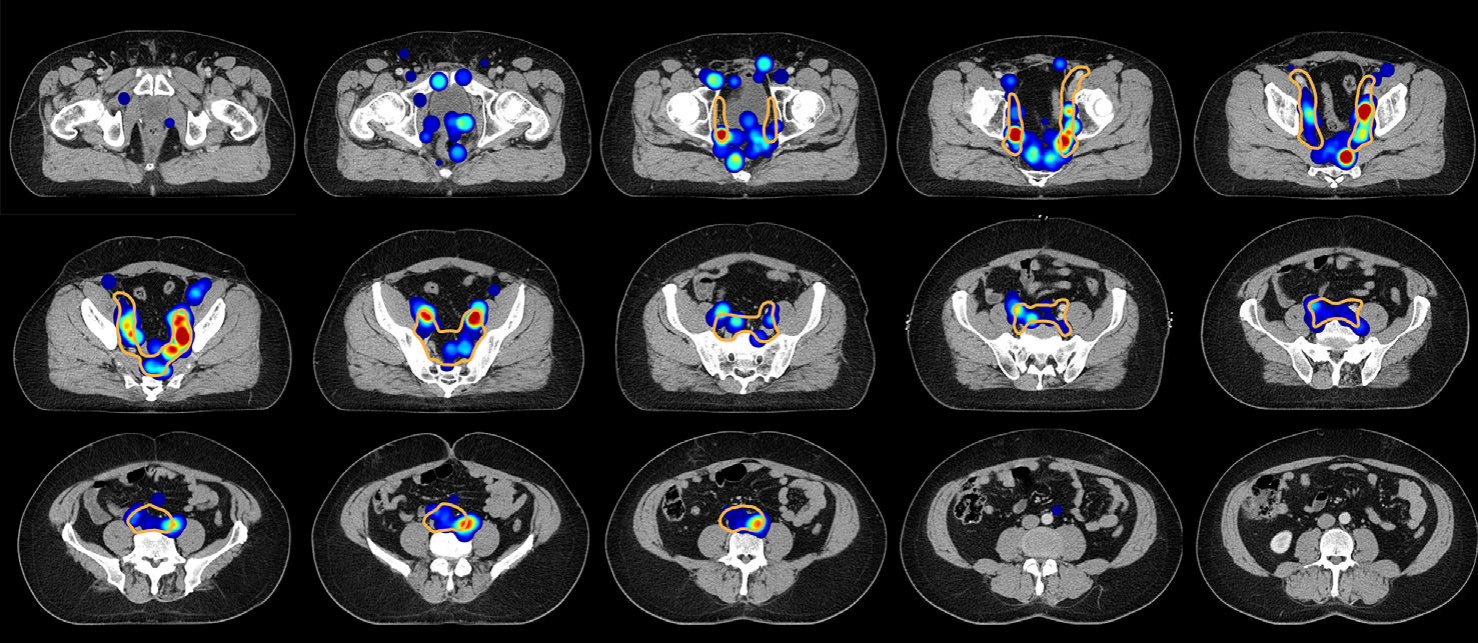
Axial slices of the created CT atlas visualize the estimated three-dimensional probability density function of pelvic nodal metastases for the whole cohort in a common reference CT. Weighting was applied so that each patient case contributed equally to the estimate irrespective of the amount of positive nodes. Slice spacing is 15 mm. Solid orange contour: Clinical target volume defined according to the recent NRG recommendation.
